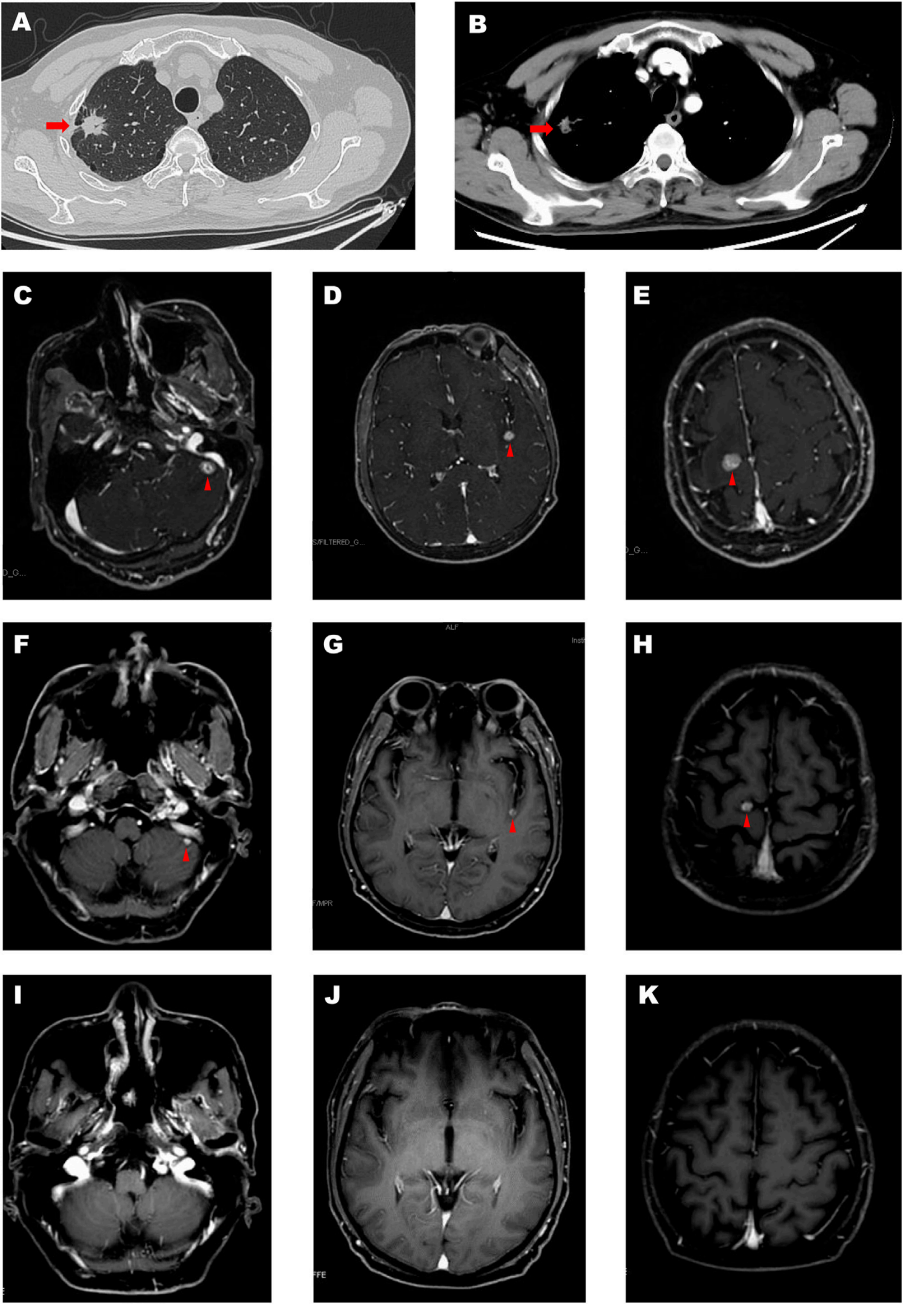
The red arrow represents the primary lesion of the lung (A,B). The red triangle represents brain metastases (C–H). And the enhanced MRI showed complete response of brain metastases (I–K).
